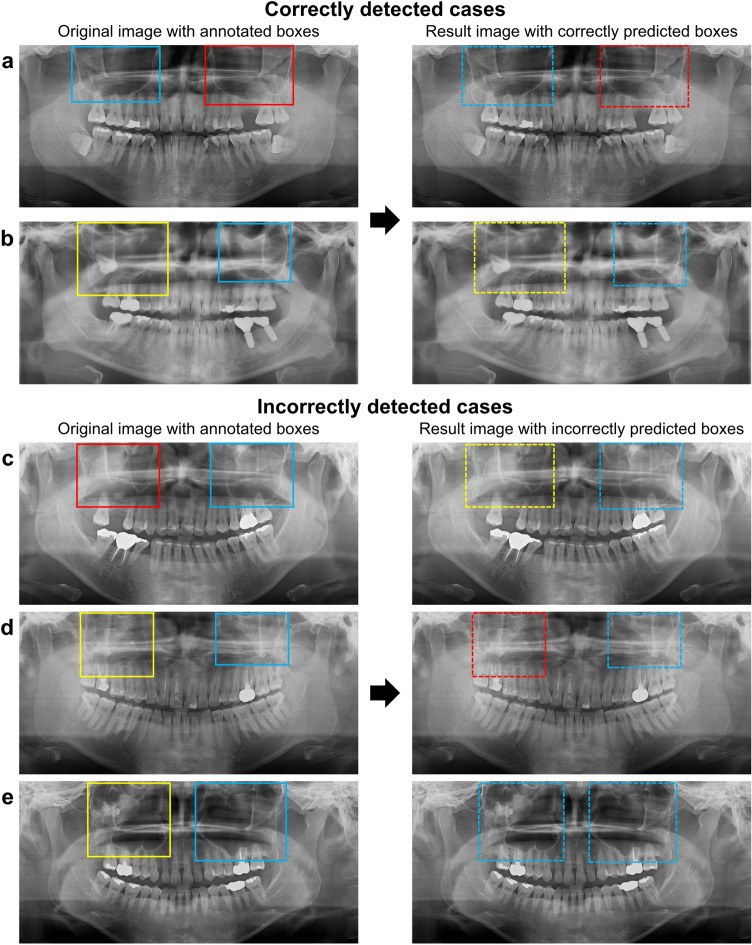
Examples of sinuses classified by the model. The left side of each case is the original panoramic radiograph with annotated boxes (solid line) and the right side of each case is the resulting panoramic radiograph with predicted boxes (dotted line) in a different color for each class (healthy: blue, retention pseudocyst: red, cyst or tumor: yellow). Examples of sinuses detected by the model with correct classes (a,b). Examples of sinuses detected by the model with incorrect classes (c–e). In the right maxillary sinuses, a retention pseudocyst was incorrectly predicted as a cyst or tumor (c), a cyst or tumor was incorrectly predicted as a retention pseudocyst (d), and a cyst or tumor was incorrectly predicted as healthy (e).
